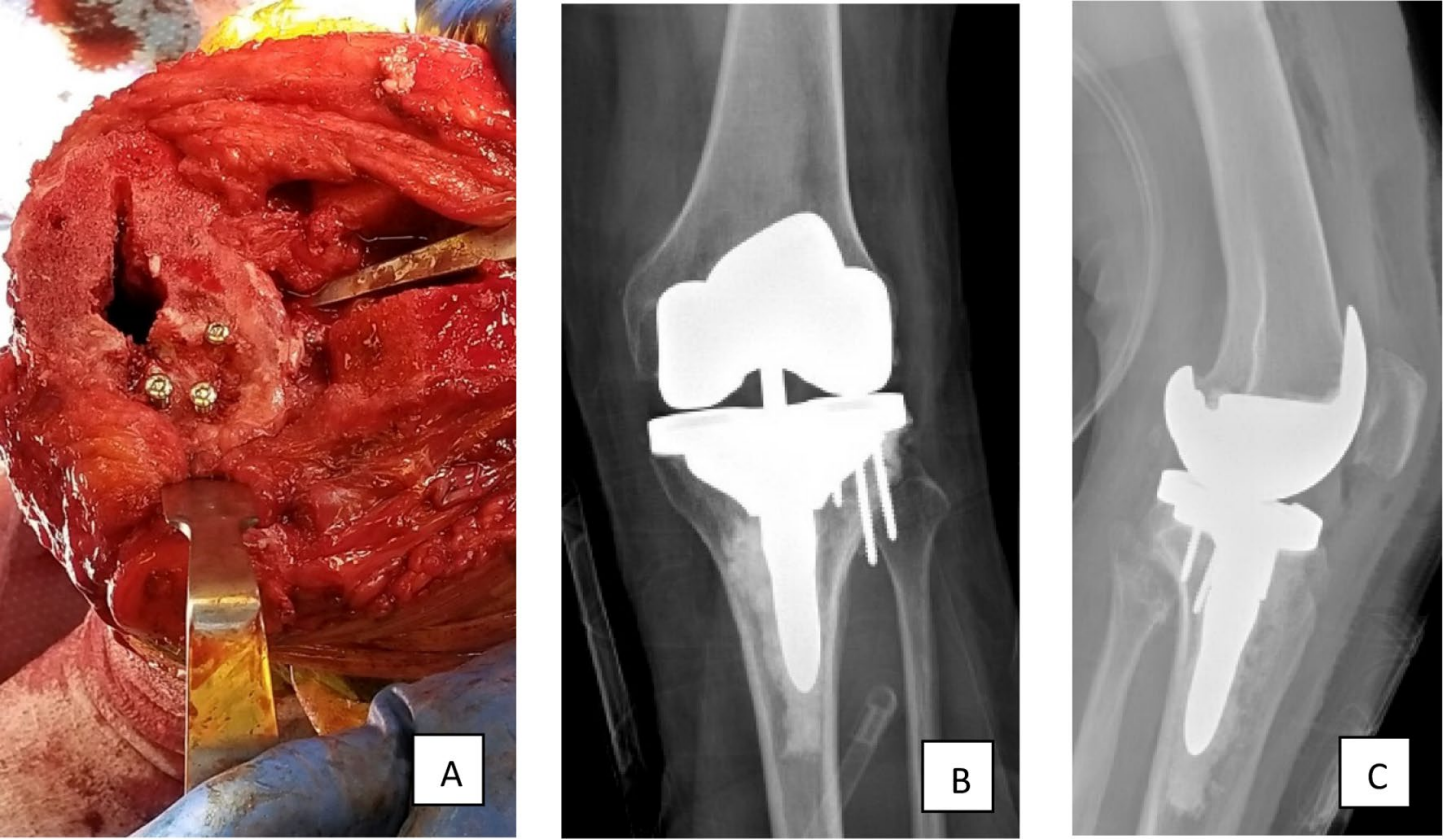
A Gross anatomy and B, C radiographic imaging of fixation screws with rebar technique in the tibial plateau
